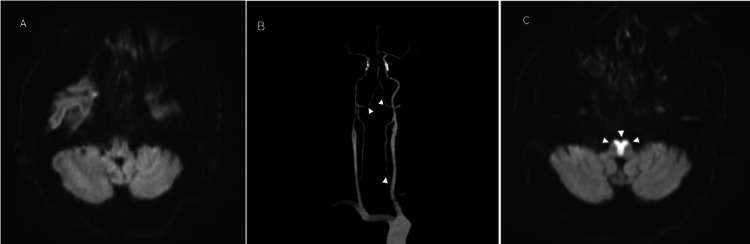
Imaging findings on Day 1 and Day 2 (A) DWI-MRI on Day 1 showing no acute ischemic lesion observed in the medulla oblongata (early DWI-negative window); (B) Head and neck CTA showing severe vertebral artery stenosis (arrowheads); (C) DWI-MRI on Day 2 showing bilateral paramedian medullary infarcts (arrowheads) presenting the classic BMMI “heart sign” imaging feature. MRI: magnetic resonance imaging; DWI: diffusion-weighted imaging; CTA: computed tomography angiography
